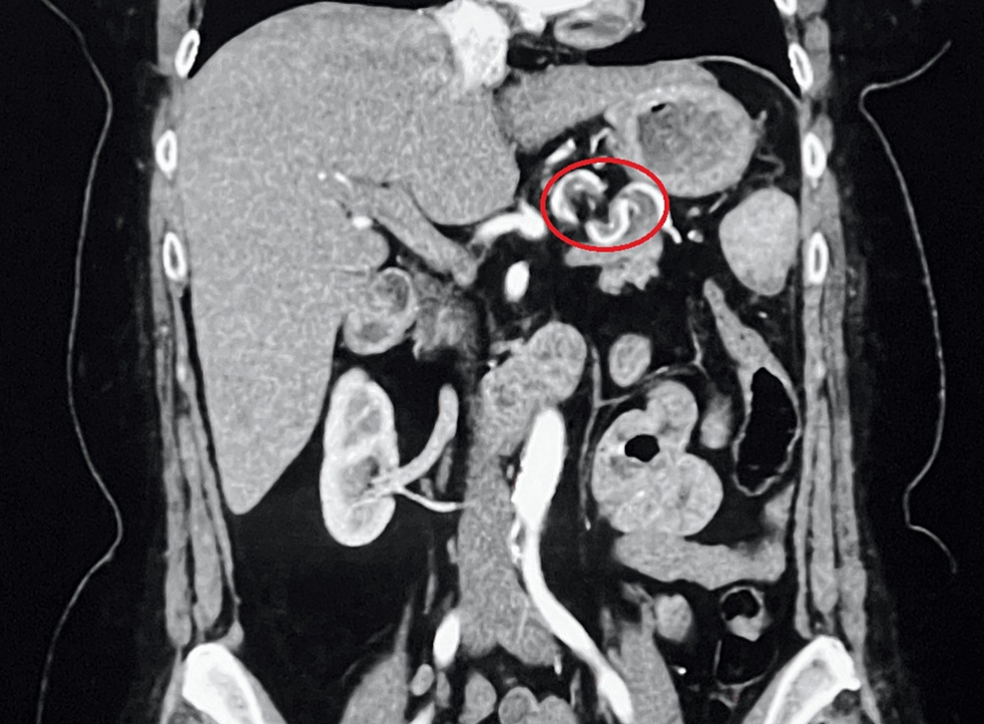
Representative image of a CTA scan of the abdomen showing an extensive filling defect in the splenic artery indicating a thrombus. Area within the red circle indicates the location of the thrombus.
